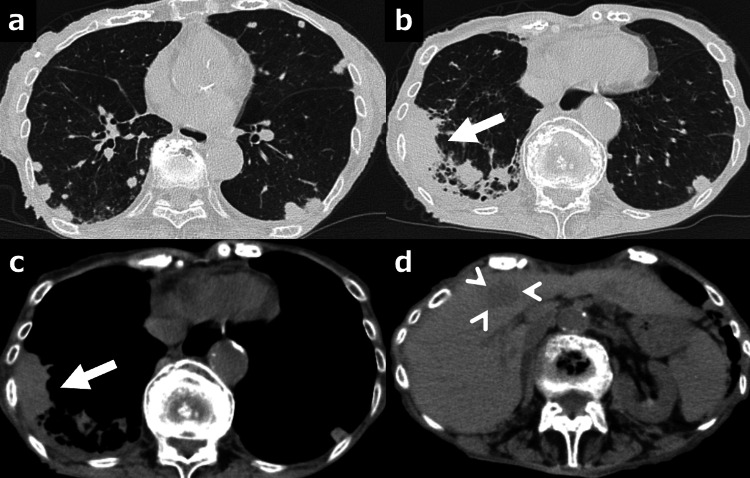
CT on final admission CT showed mass shadows in bilateral lung fields (a), a mass shadow (arrow) just below the pleura (b, c), and a mass shadow (arrowheads) in S4 of the liver (d) CT: computed tomography
